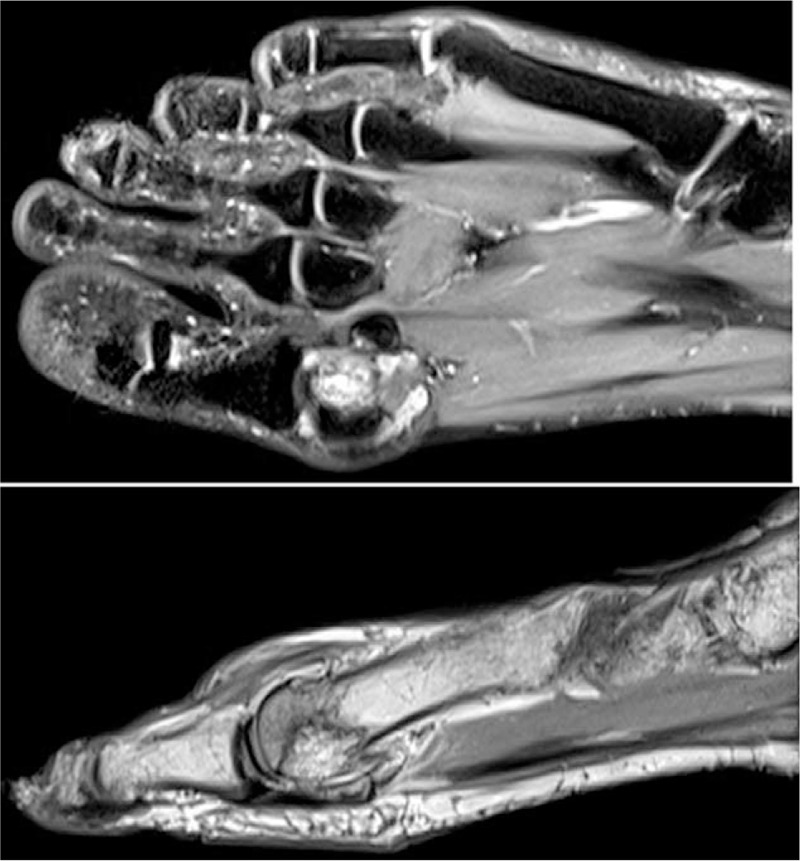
Case 2. MRI showed irregular infiltrating soft tissue mass at around of left first metatarsal-phalangeal joint causing bony destruction/erosion and osseous involvement.
